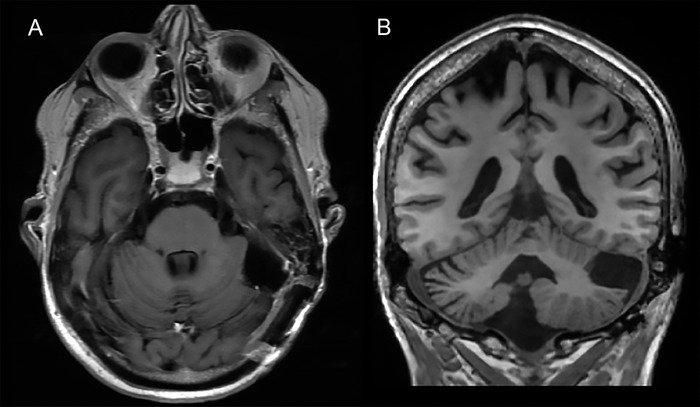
Postcontrast (A) axial and (B) coronal brain magnetic resonance images obtained at 1-year follow-up demonstrate postsurgical changes with no evidence of tumor regrowth or recurrence.
